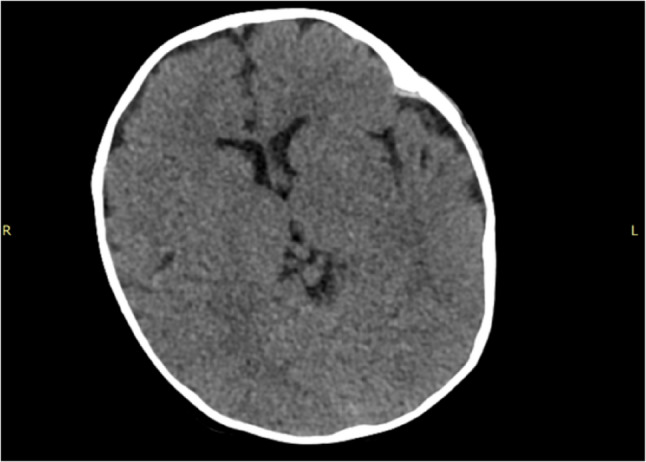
Head CT scan performed on admission showed no significant abnormalities
